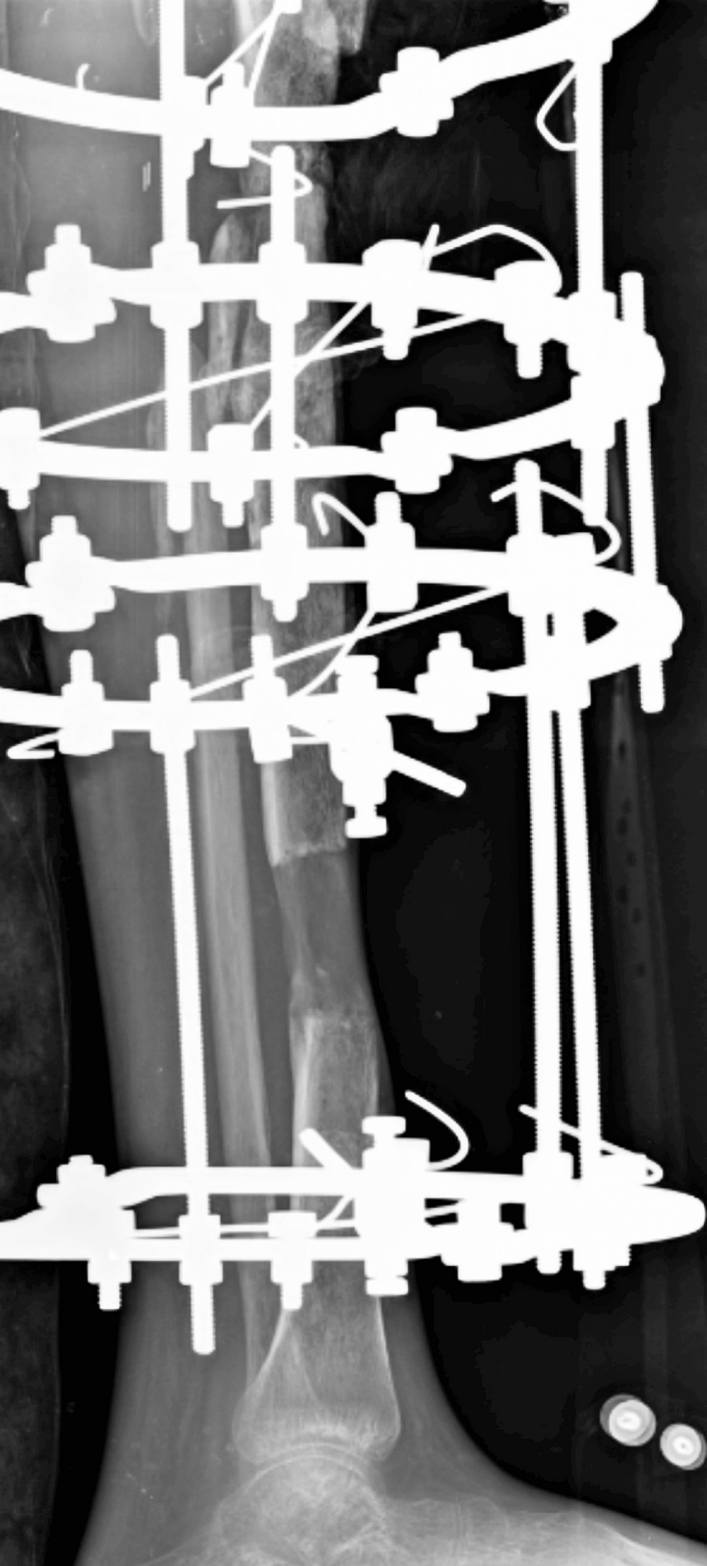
Post-operative x-ray of a compound grade 3c tibial fracture with Ilizarov fixators.
